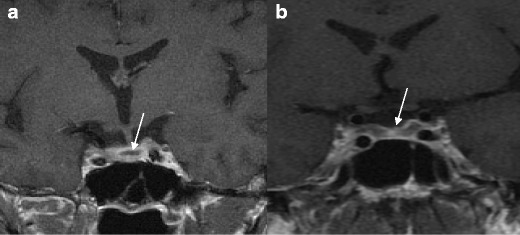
Case 4 (suspected HIV-related pituitary haemorrhage). a Coronal MRI at presentation showing likely haemorrhage within enlarged pituitary gland. b Six-month follow-up MRI showing reduction in pituitary size with concave upper border
